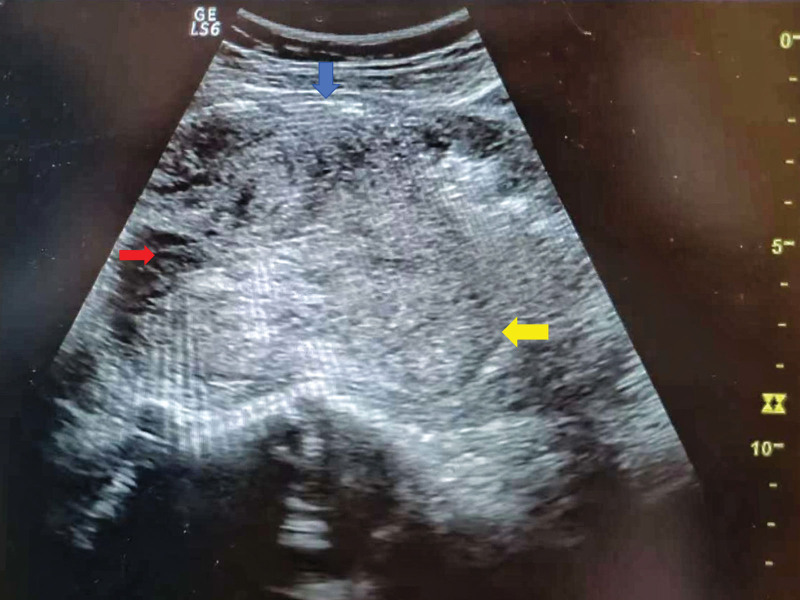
Uterine inversion-ultrasound imaging. Blue arrow: plasma layer of the anterior uterine wall; Red arrow: cupping structure formed after inversion of the uterus; Yellow arrows: fundus tissue that inverts into the uterine cavity.
